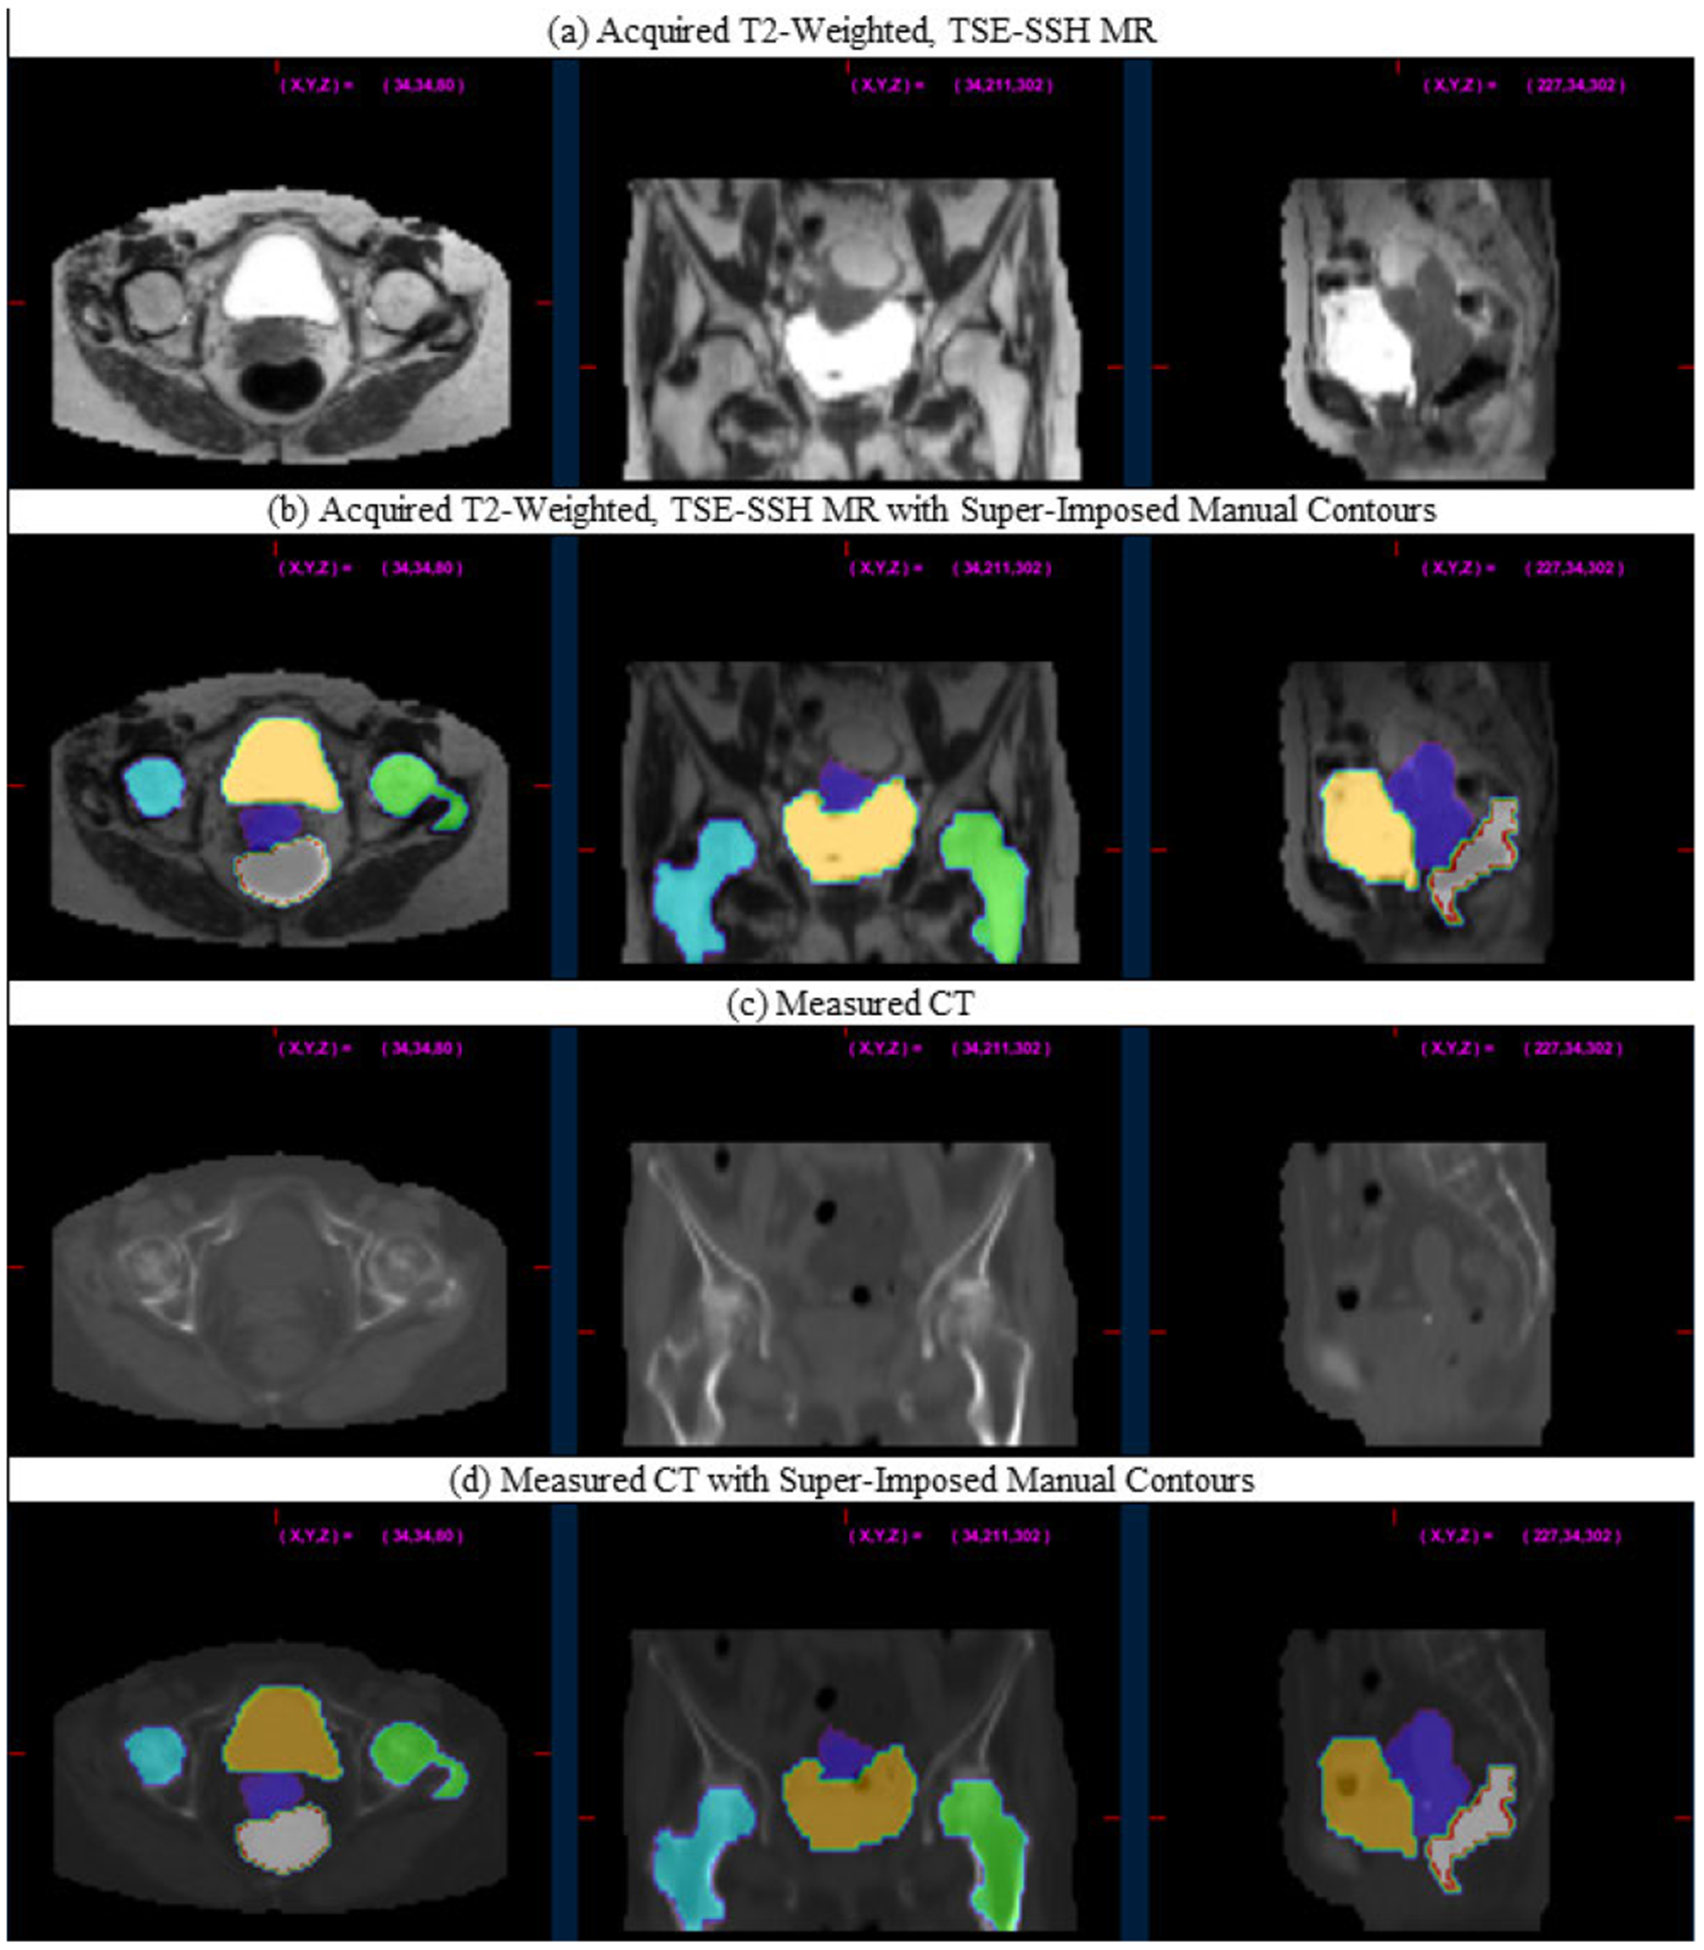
Axial, coronal, and sagittal (left to right) views of T2-weighted TSE-SSH MR (a), with the measured CT (b) after registration. Manual contours were initially drawn on the MR images (b), then projected to the CT images (d). GTV: Violet, Right Femur: blue, Left Femur: Green, Bladder: Orange, Anoerctum: Grey.
